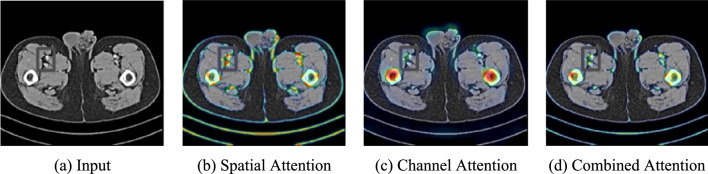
Grad-CAM visualization of the dual-feature attention mechanism on a representative LDCT image processed by our method. a Input CT image, b Spatial attention highlighting anatomical boundaries, c Channel attention focusing on texture-rich regions, d Combined attention showing final feature weighting. Warmer colors indicate higher attention weights
